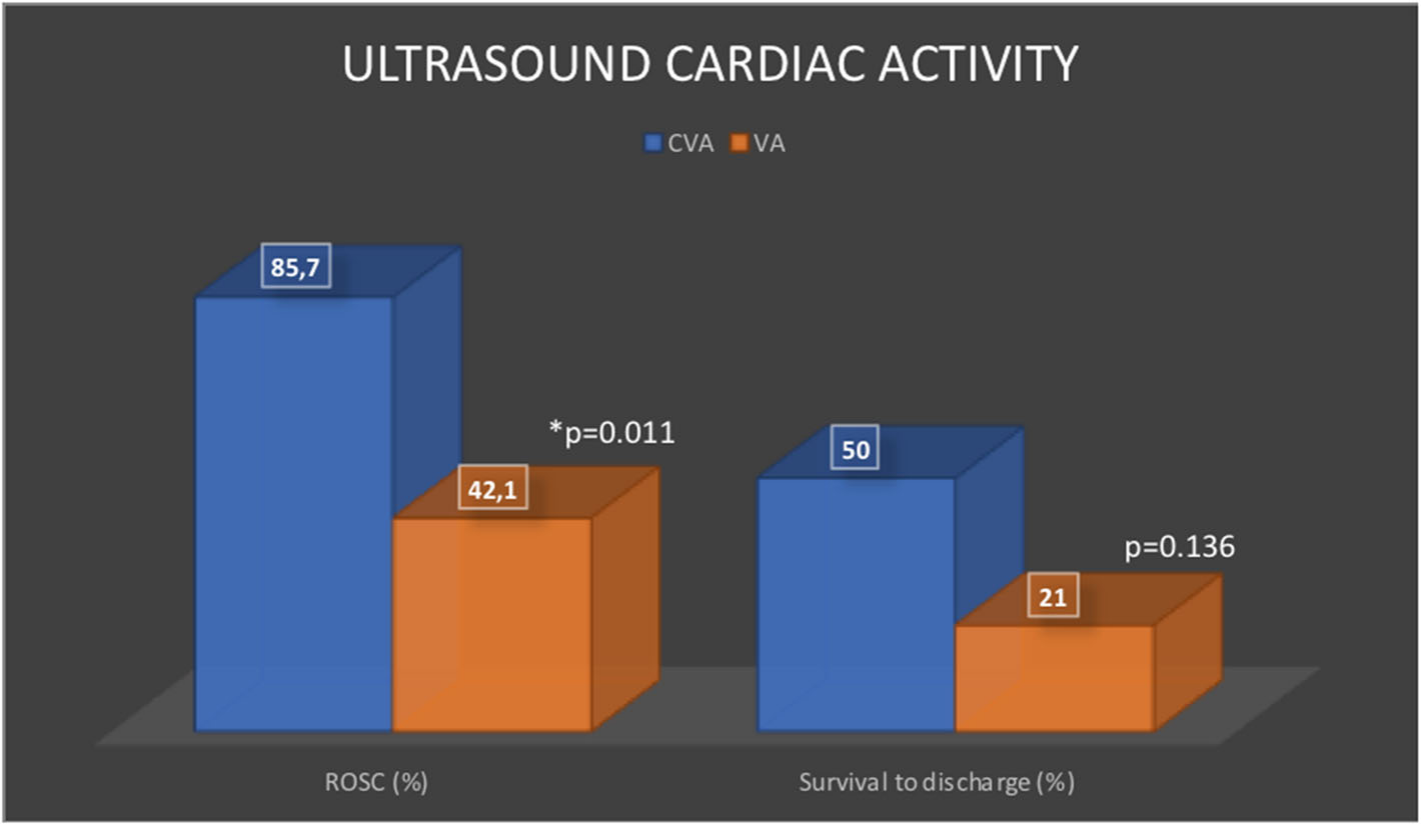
Ultrasound cardiac activity. ROSC, return of sustained circulation; CVA, cardiac and valvular activity; VA, valvular activity only
